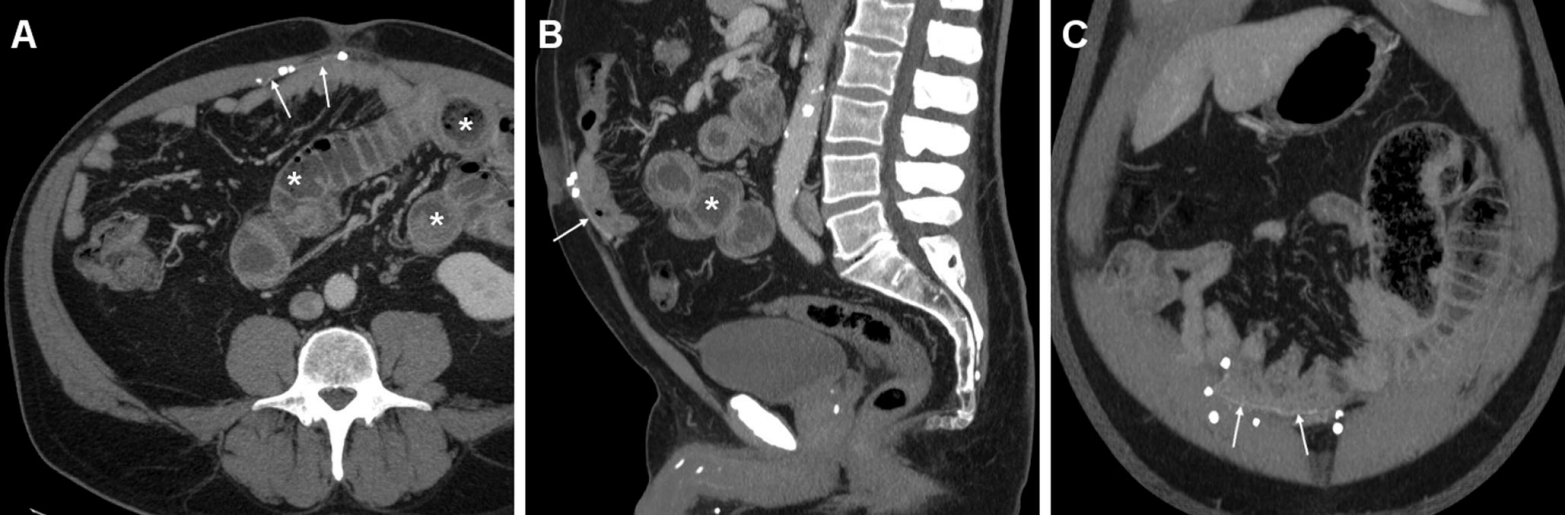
Usual imaging appearance of expanded polytetrafluorethylene (ePTFE) PM following recent laparoscopic repair of a ventral incisional hernia in a 70-year-old overweight male, suffering from postoperative abdominal pain and vomiting. Axial (a), sagittal (b) contrast-enhanced CT images and coronal maximum-intensity projection (MIP) reconstruction (c) showed the moderately hyperattenuating PM (thin arrows), fixed by metallic sutures, without abnormal collections. Note distended small bowel loops (*) with intraluminal fluid consistent with clinical diagnosis of postoperative intestinal obstruction, which required laparotomic surgery including redo hernia repair
